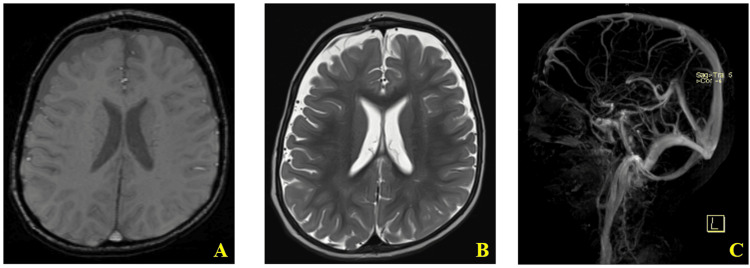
Brain MRI images of the patient at the presentation A. Normal brain MRI (axial plane, T2 flair).  B. Normal brain MRI with contrast (axial plane) showing the non-enhancing white matter. C. Normal brain magnetic resonance venography (MRV) with normal appearance of the major venous sinuses and superficial veins with no evidence of thrombosis, obstruction, filling defects, or infiltration.
